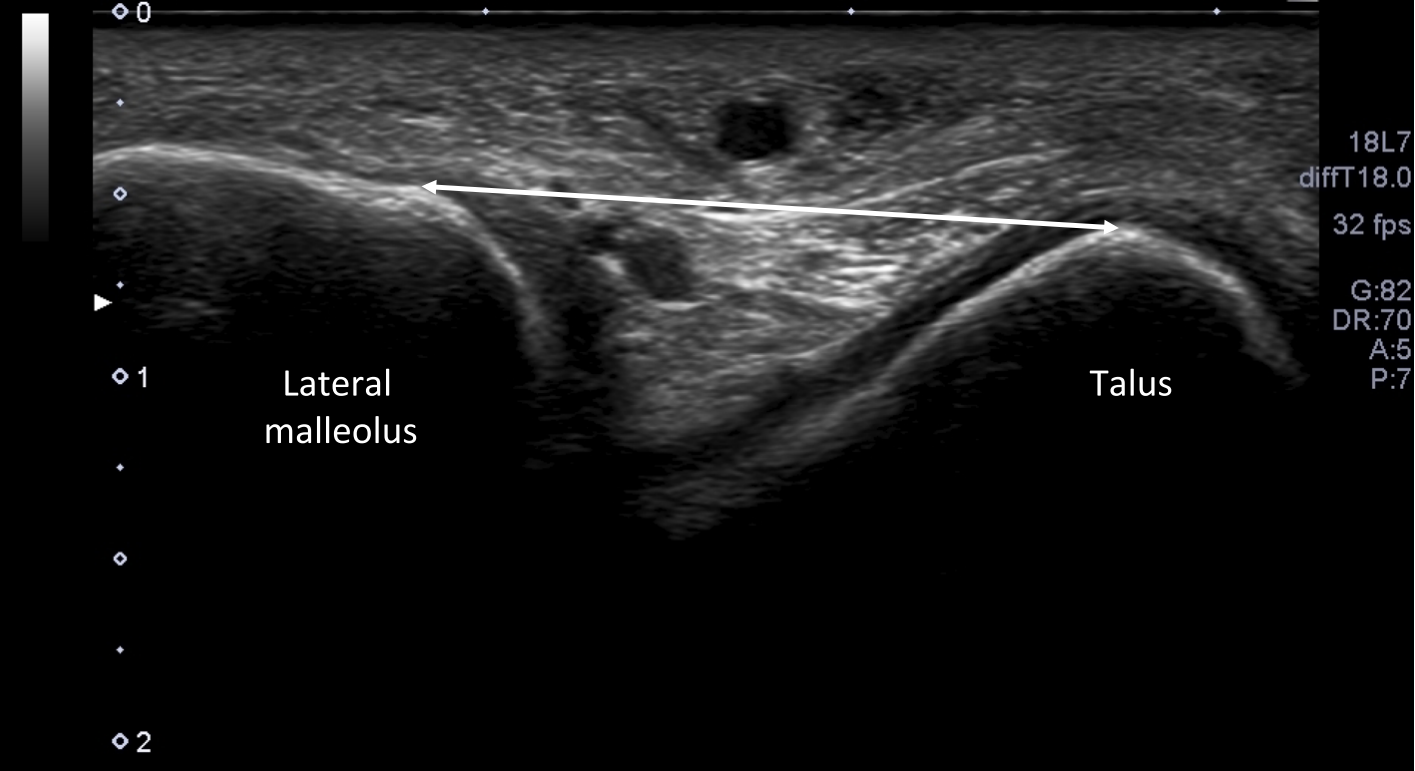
Ultrasound image for measurement of the anterior talofibular ligament length. The ultrasound image captured directly over the anterior talofibular ligament origin and insertion allows the examiner to use a straight line measurement tool to draw a line from the anterolateral aspect of the lateral malleolus to the talus, points that correspond to the anatomic attachment sites of the ligament
